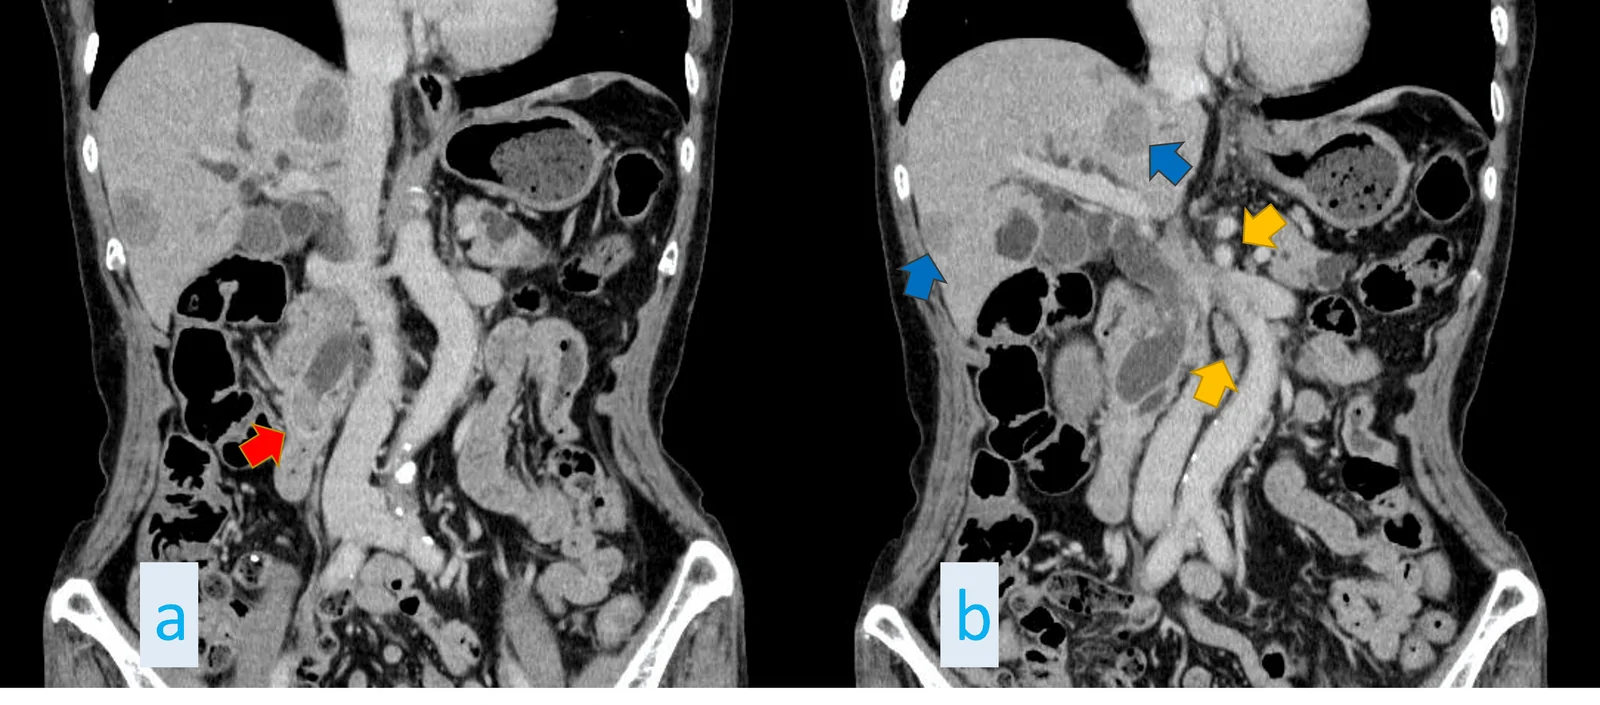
Contrast-enhanced abdominal computed tomography. (a) Contrast-enhanced abdominal computed tomography revealed a 25-mm mass at the ampulla of Vater, showing persistent enhancement from the arterial to equilibrium phases (red arrow), accompanied by dilation of the bile duct and main pancreatic duct. (b) Para-aortic lymph node metastasis (yellow arrow) and multiple hepatic metastases (blue arrows) were observed.
